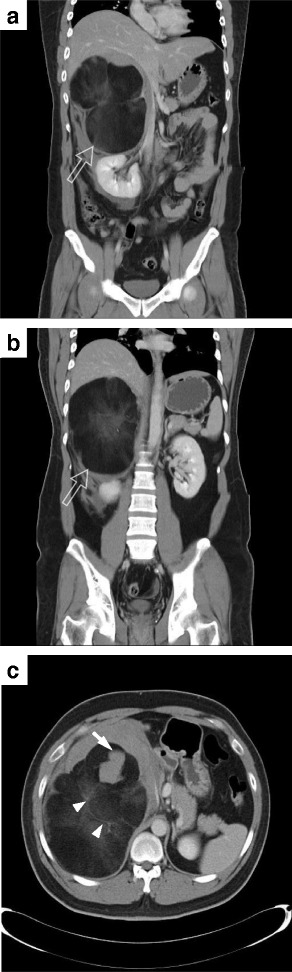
CT of abdomen with contrast showed a huge retroperitoneal majorly fat-content mass (*open arrow*) over right suprarenal (a & b) with ruptured hemorrhage (*arrow*), and interspersed with enhancing soft tissue components, as smoky appearance (*arrowhead*) (c)
